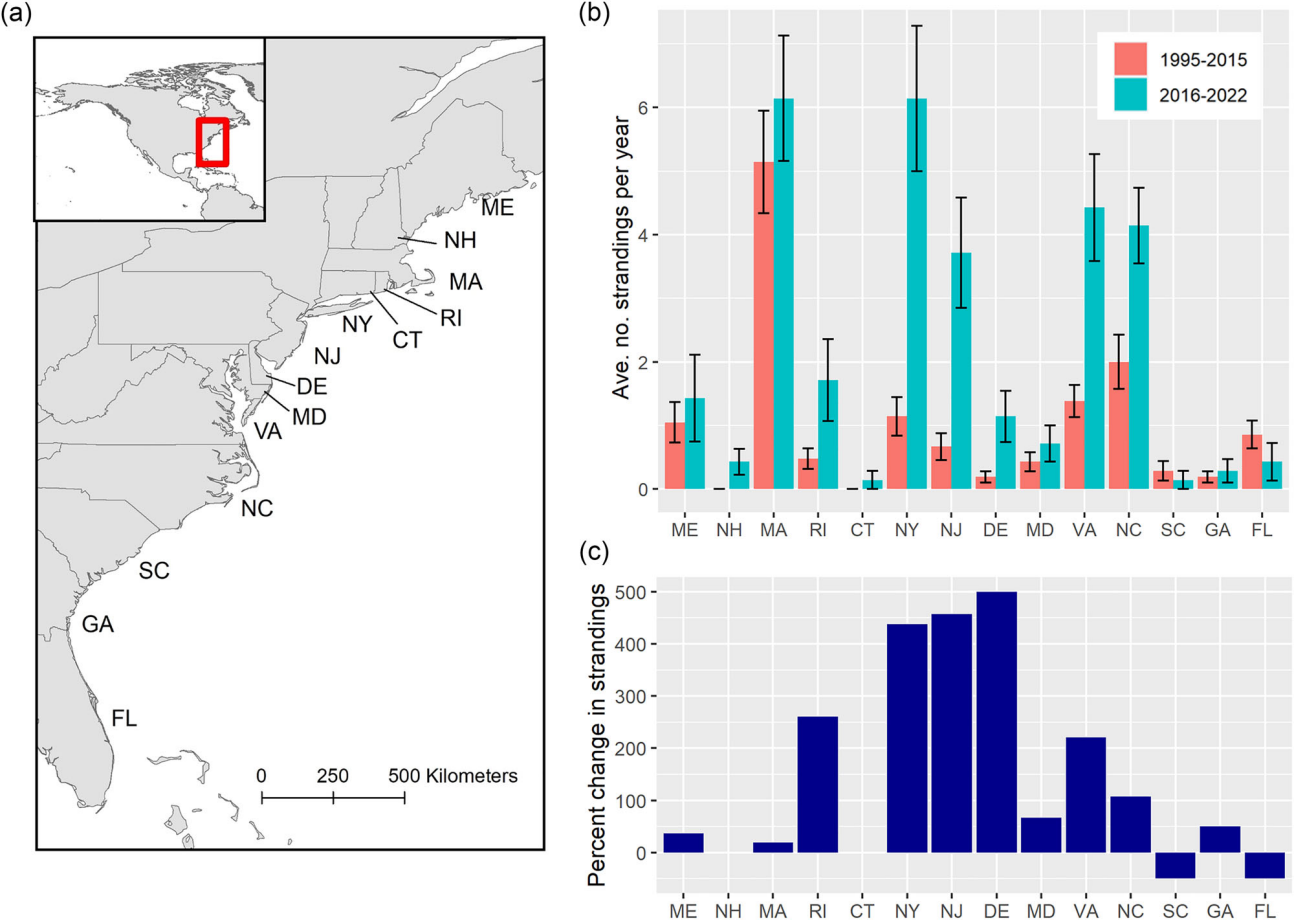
(a) Location of states along the East Coast of the United States, (b) average number of humpback whale strandings (+/‐ SE) per year by state prior to 1995−2015 and during the unusual mortality event (UME) (2016−2022), and (c) percent change in humpback whale strandings by state during UME in relative to previous years (1995−2015) (ME, Maine; NH, New Hampshire; MA, Massachusetts; RI, Rhode Island; CT, Connecticut; NY, New York; NJ, New Jersey; DE, Delaware; MD, Maryland; VA, Virginia; NC, North Carolina; SC, South Carolina; GA, Georgia; FL, Florida).
